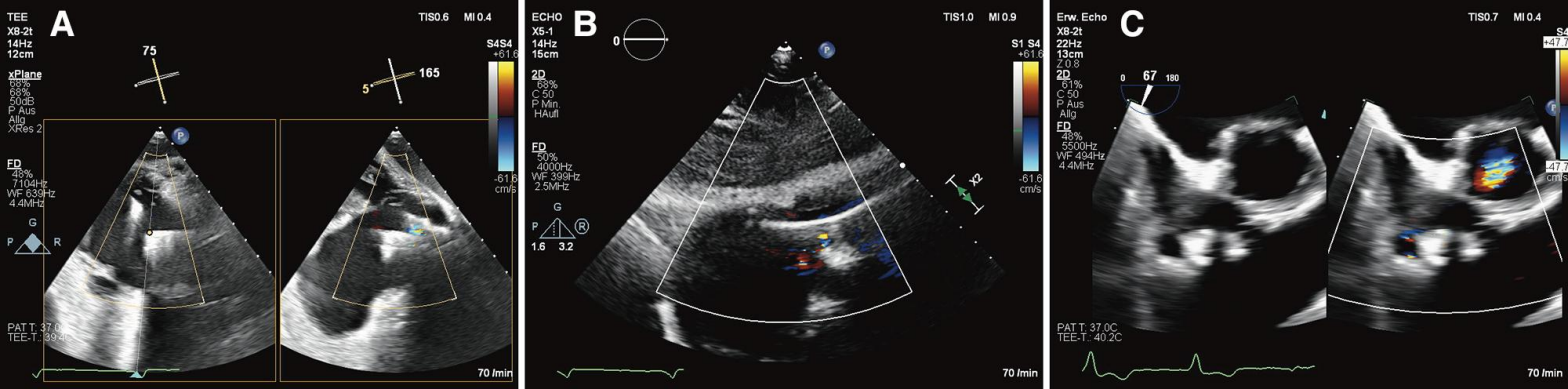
Immediate procedural result showing a minimal TR between Pascal Ace® (Edwards Lifesciences, Irvine, CA) and right ventricular lead (*A*). After 1 month, a stable result with only mild TR in subcostal view (*B*) is documented and in the inflow–outflow view (transoesophageal echocardiogram) at 1-year follow-up (*C*).
